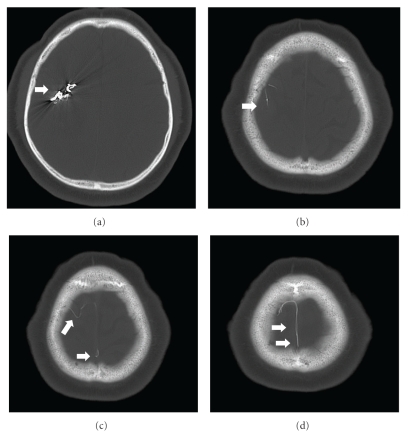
Bone windows of CT of the head after first embolization, showing high attenuation embolic material (Onyx) in the nidus of the AVM (a) as well as extension through the draining vein and a thin strand in the superior sagittal sinus.
